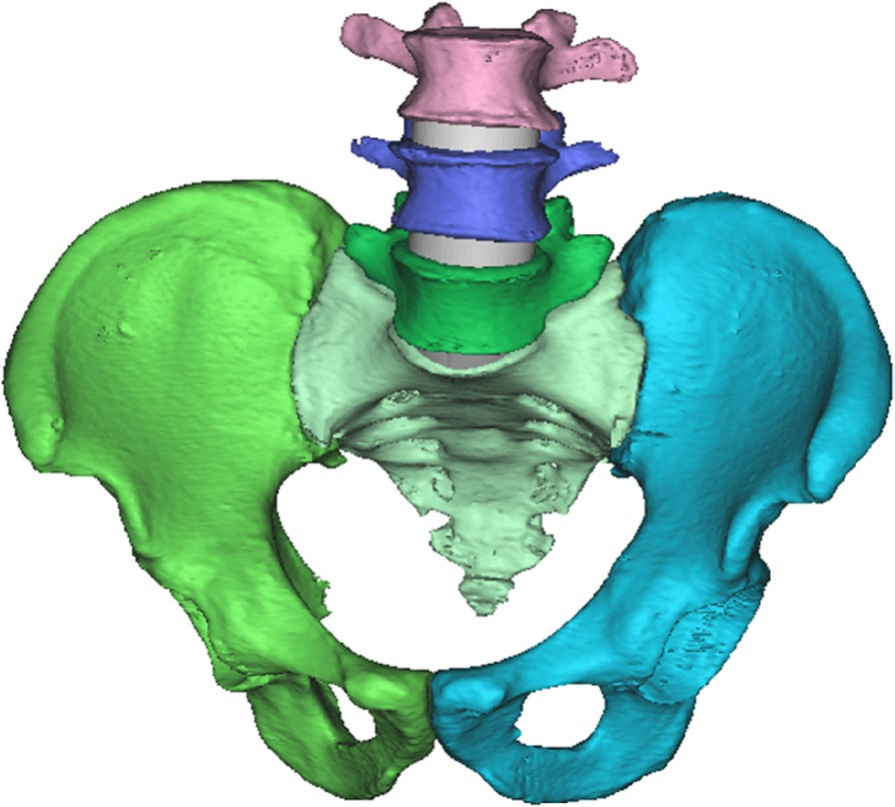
Individual pelvic components generated from CT data
